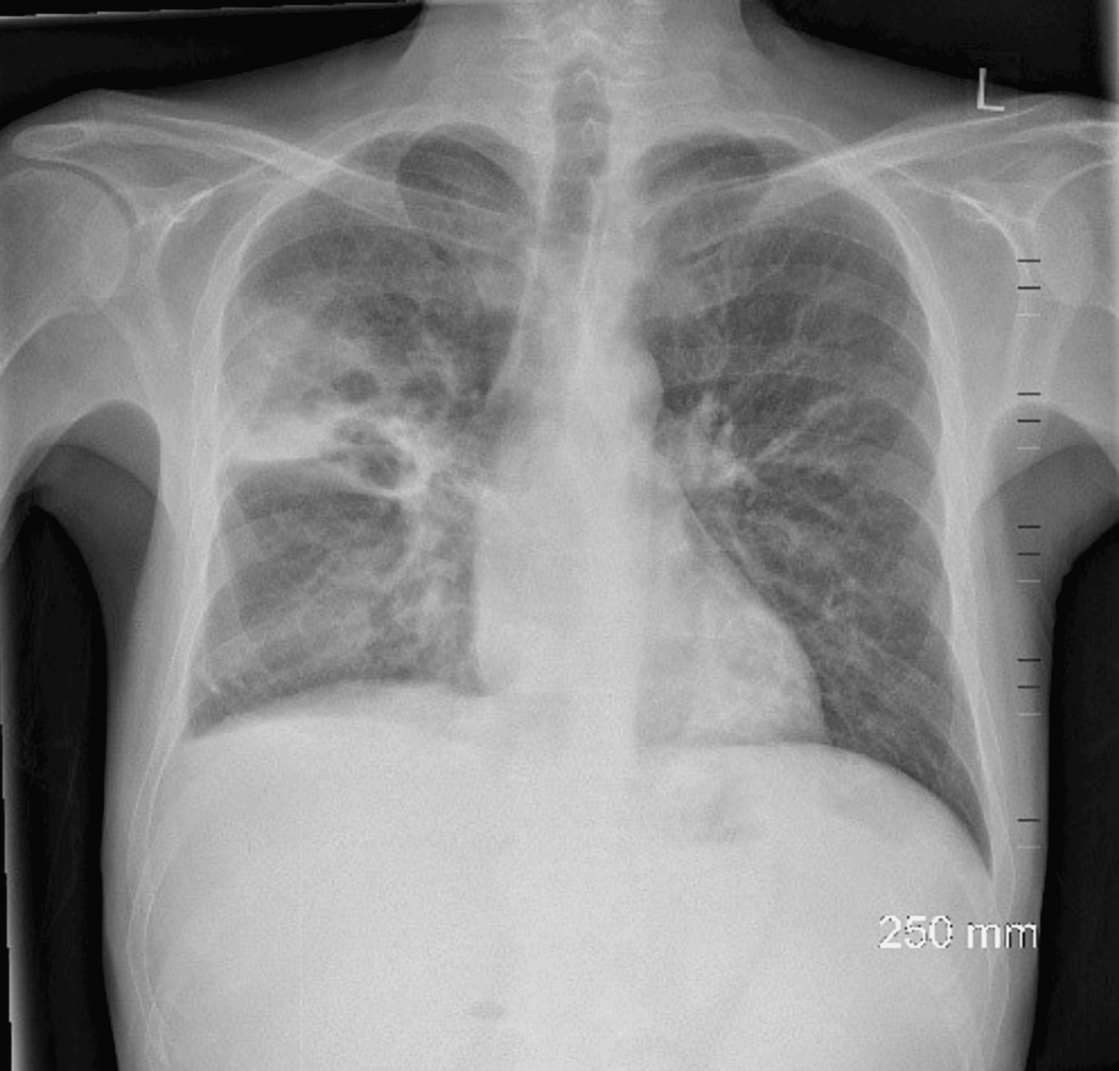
Chest X-ray on admission
